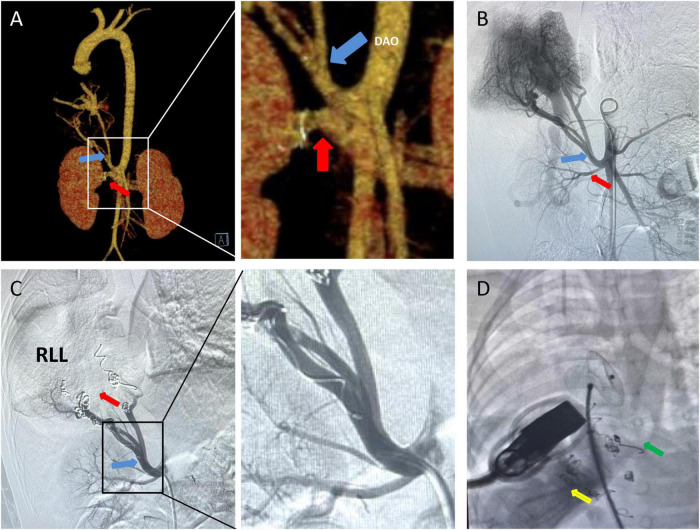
CT 3D reconstruction and Aortic DSA: (A,B) A isolates the pulmonary supplying artery (blue arrow) and the renal artery (red arrow) to the abdominal aorta, and isolates the pulmonary supplying artery by approximately 4 mm. (C) Nine platinum coils were placed in the isolated pulmonary supplying artery (blue arrow), and no contrast agent entered the distal end of the infarct (red arrow). (D) Placement of atrial septal defect occluder (green arrow) under co-guided echocardiography (yellow arrow) and DSA. DAO, decending aorta; RLL, right lower lobe.
